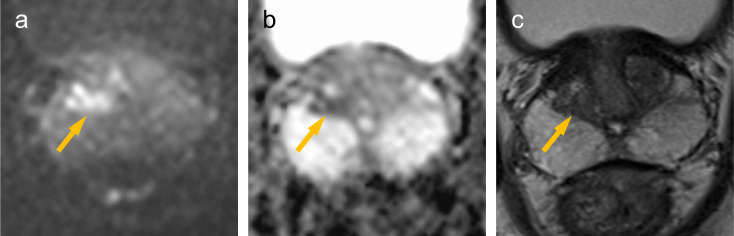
MRI (3.0T). There is a nodule in the right transition zone (→). The nodule shows hyperintense signal on DWI (a), hypointense signal on ADCmap (b). But the nodule is not identifiable on T2WI, since the nodule shows equal signal to the surrounding transition zone (c).
